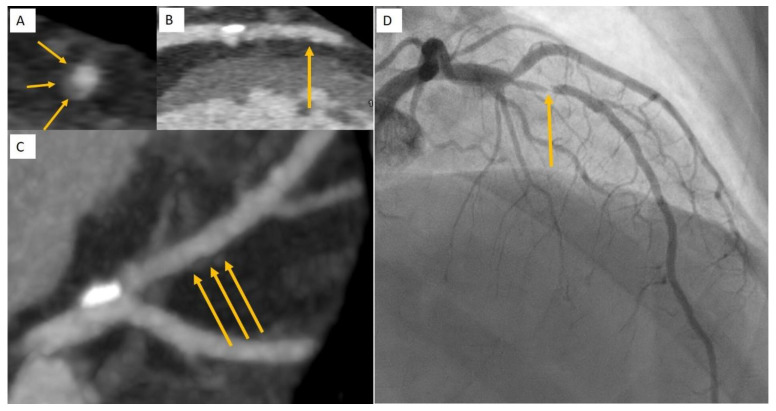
Subclinical atherosclerosis to clinical atherosclerosis. Subclinical atherosclerosis was detected during a CCTA to investigate new onset of reduced left ventricular ejection fraction of 41%. A diagnosis of non-ischemic cardiomyopathy was determined following CCTA and cardiac MRI. A mild lesion in the mid LAD was noted at CCTA to have positive remodelling (A), low attenuation plaque and spotty calcification (B,C) on multiplanar reformat (A,B) and maximum intensity projection (C). One month later the patient presented with a non-ST-elevation myocardial infarction due to an acute plaque event of the mid LAD plaque (D).
